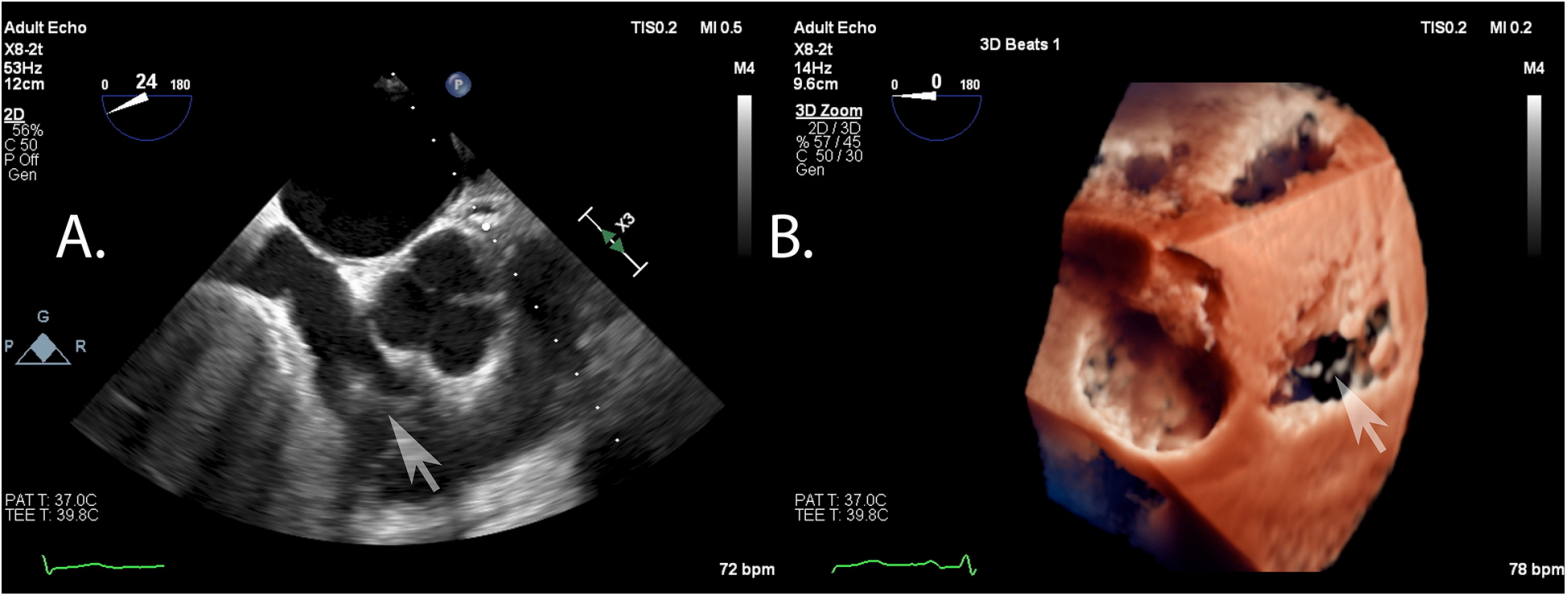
Example of typical artifacts precluding a proper visualization of the tricuspid valve: shadowing from the intra-atrial septum and signal attenuation as seen in the *en face* view of two- (A) and three-dimensional (B) echocardiography.
